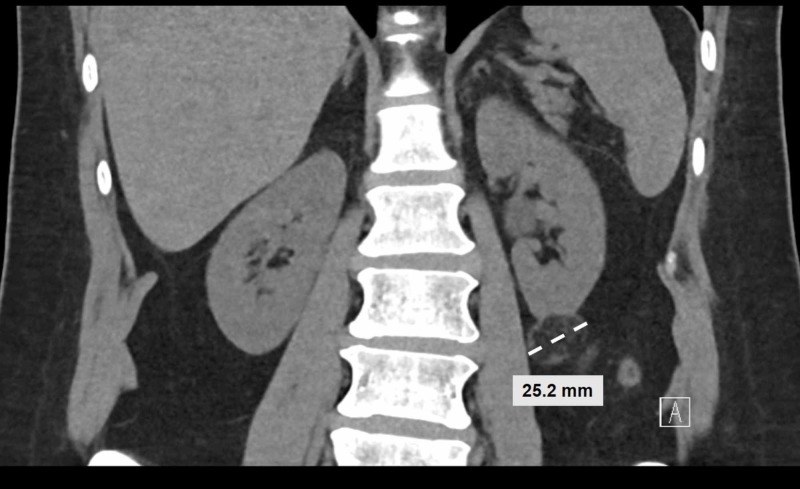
Angiomyolipoma identified from CT imaging
